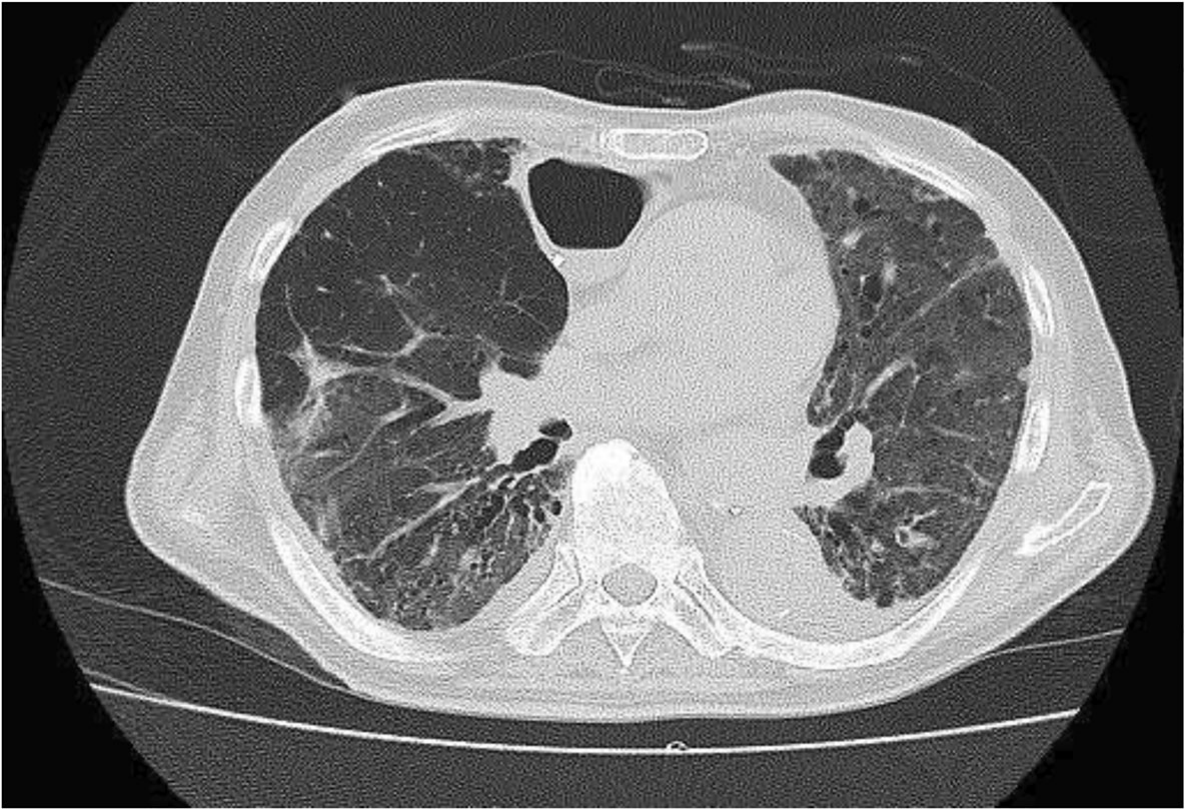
Computed tomography image showing bilateral pulmonary fibrosis
